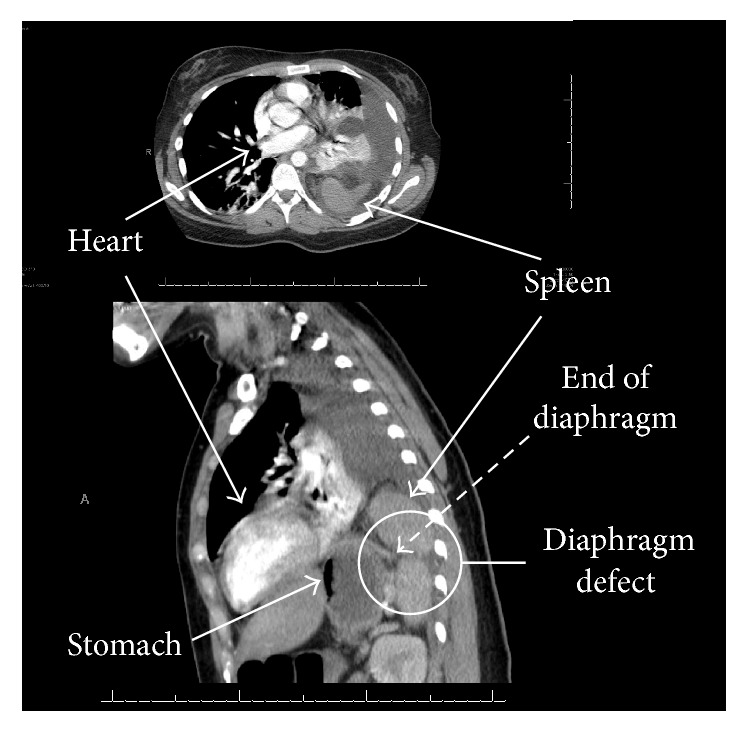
Thoracic CT images showing diaphragm rupture with herniated spleen.
